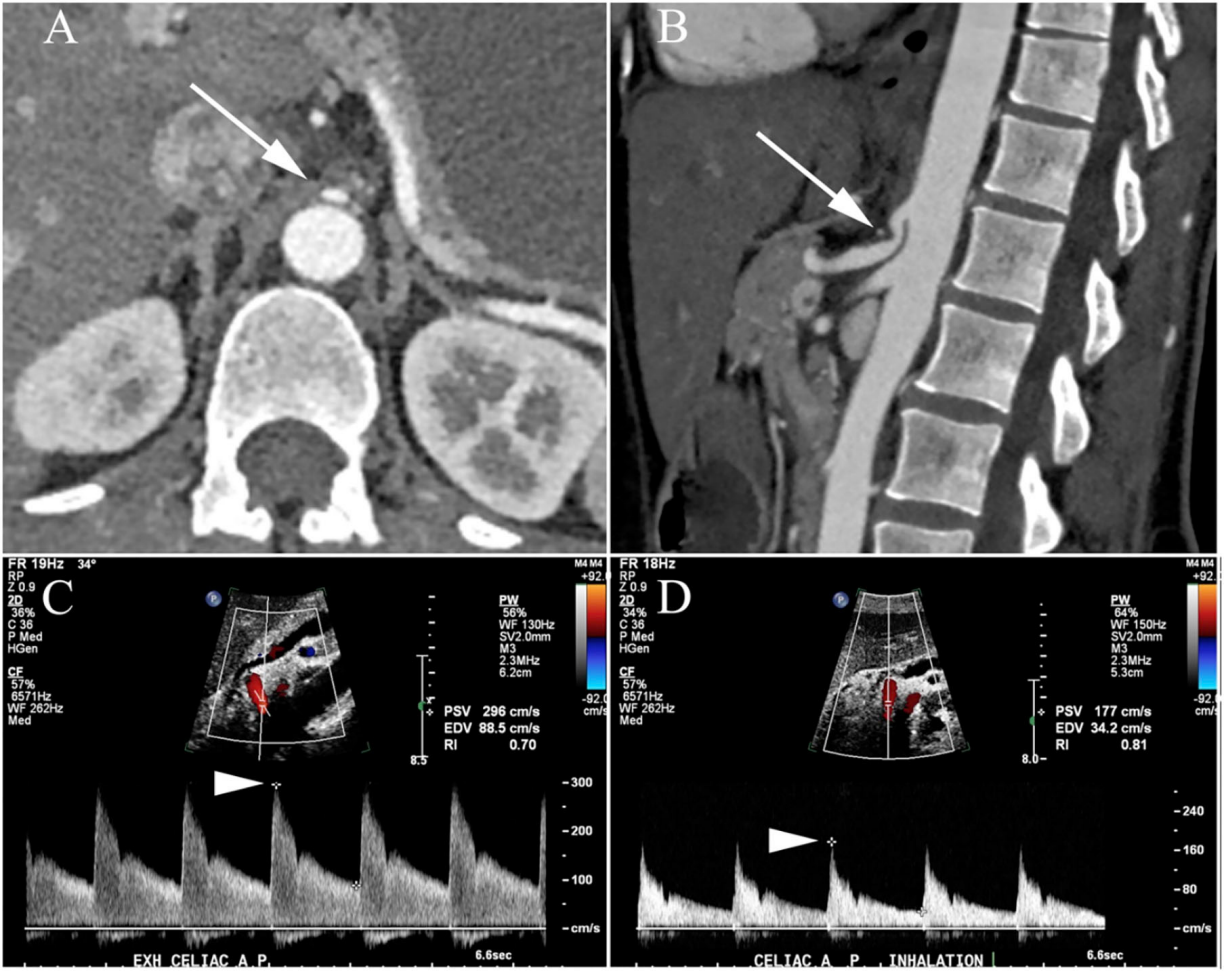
Patient with neurogenic median arcuate ligament syndrome (nMALS). Axial (A) and sagittal (B) CTA images demonstrate severe narrowing of the celiac artery origin (arrow) related to extrinsic compression by the median arcuate ligament. Duplex ultrasound demonstrated normal arterial flow during expiratory ventilation with a peak systolic velocity (PSV) of 286 cm/s (arrowhead, C) but marked flow impairment during inspiration with PSV of 177 cm/s (arrowhead, D) equivalent to >70% stenosis and hemodynamic compromise.
